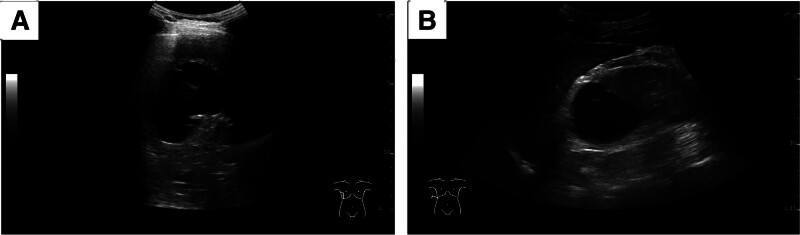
Follow-up US conducted one year after surgery indicated a significant reduction in the cyst present in the liver and kidney. (A) Liver US reveals a significant decrease in the size of a previously giant liver cyst following US-guided drainage and sclerotherapy. (B) Considerable reduction of a large renal cyst on US one year after US-guided drainage and sclerotherapy. US = ultrasound.
